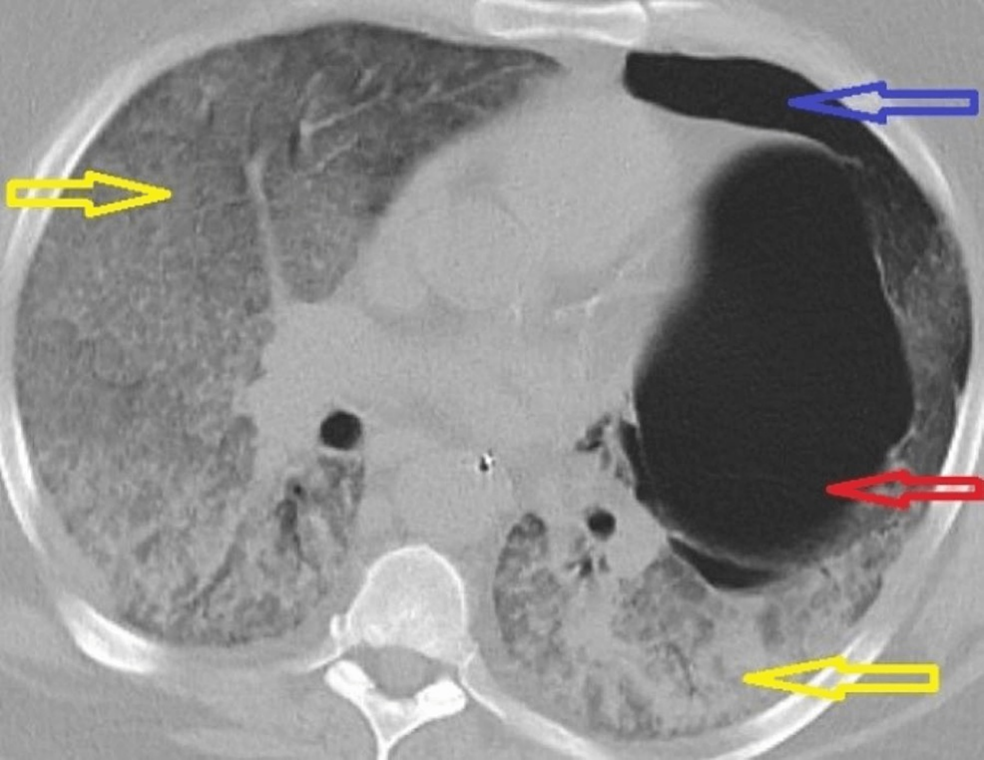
Computed tomography of the chest without contrast. Diffuse bilateral airspace consolidation (yellow arrows), existing pulmonary bleb (red arrow), and unresolved pneumothorax (blue arrow) were noted.
